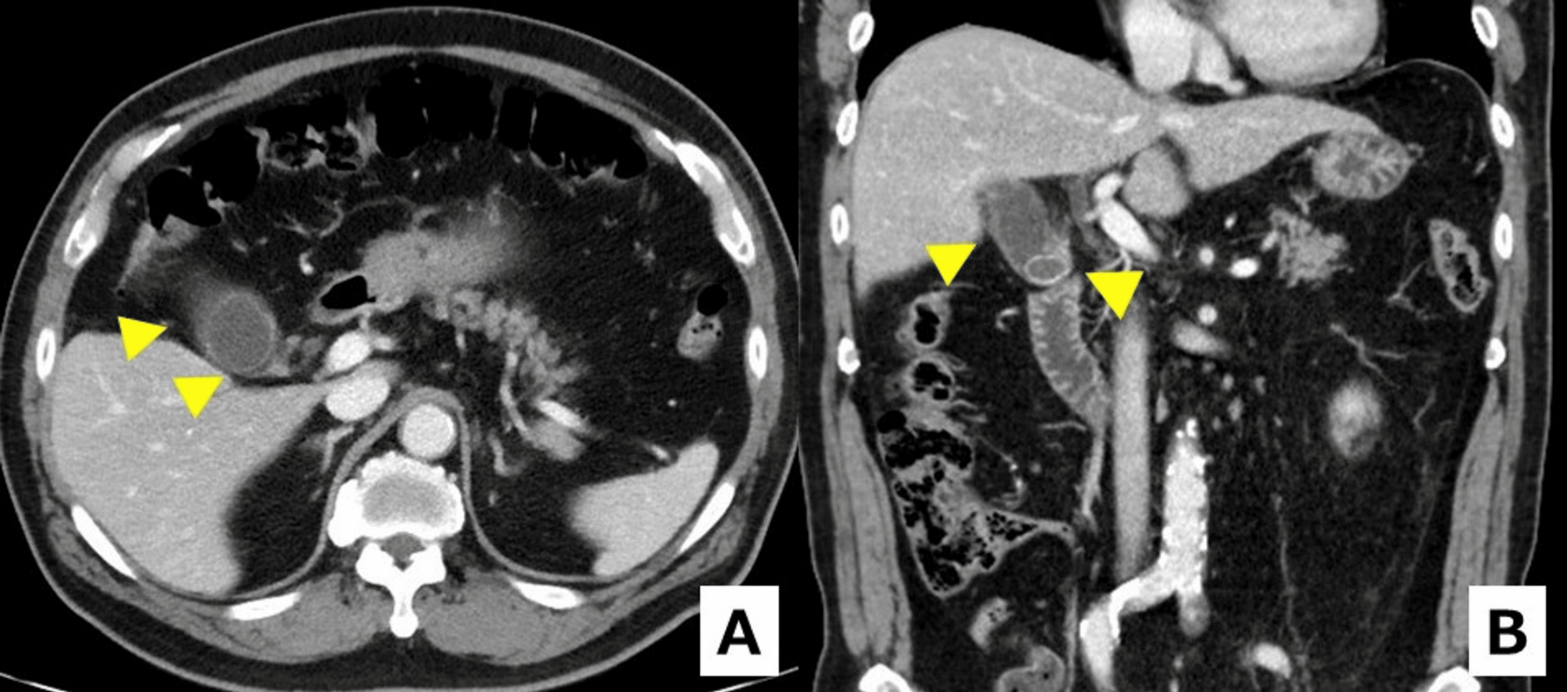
Admission contrast-enhanced CT demonstrating acute calculous cholecystitis (A) Axial and (B) coronal images show gallstones within the gallbladder, mural thickening, and pericholecystic inflammatory changes (arrowheads). CT: computed tomography
